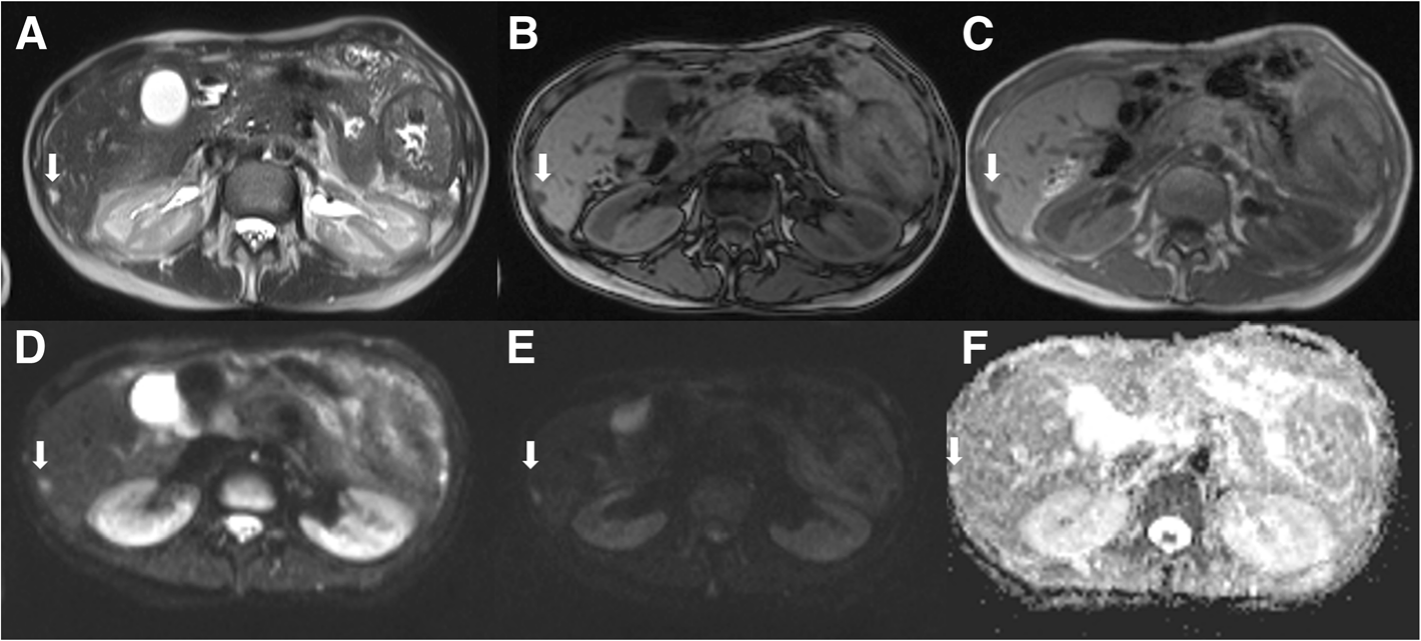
Woman 72 year with colon cancer. Follow up post chemotherapy treatment in presurgical setting. Sub-capsular metastasis (arrow) on VI hepatic segment: in a (HASTE T2-W) the lesion appears more hyperintense. In b and c (T1-W in-out phase sequences) the metastasis (arrow) is hypointense. In DWI sequences (d: b50 s/mm^2^; e: b800 s/mm^2^; f: ADC map), the lesion (arrow) shows a restricted diffusion for all b values. The ADC value is 1.40 × 10–3 mm^2^/s
